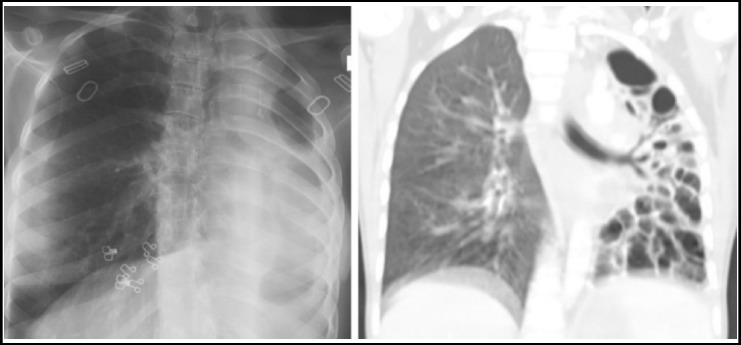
Chest X ray shows loss of lung volume with multicystic area on the left along with ipsilateral mediastinal shift. Corresponding CT chest with lung window settings of the same patient shows cicatrization collapse of the left lung.
